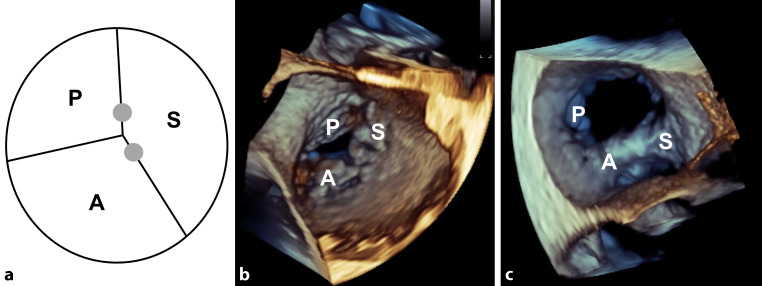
a Tricuspid valve (TV) anatomy and most common clip locations (*grey dots*). Three-dimensional echocardiographic view of the TV b before and c after implantation of two clips on the anterior and septal leaflets. *A* anterior leaflet, *P* posterior leaflet, *S* septal leaflet
